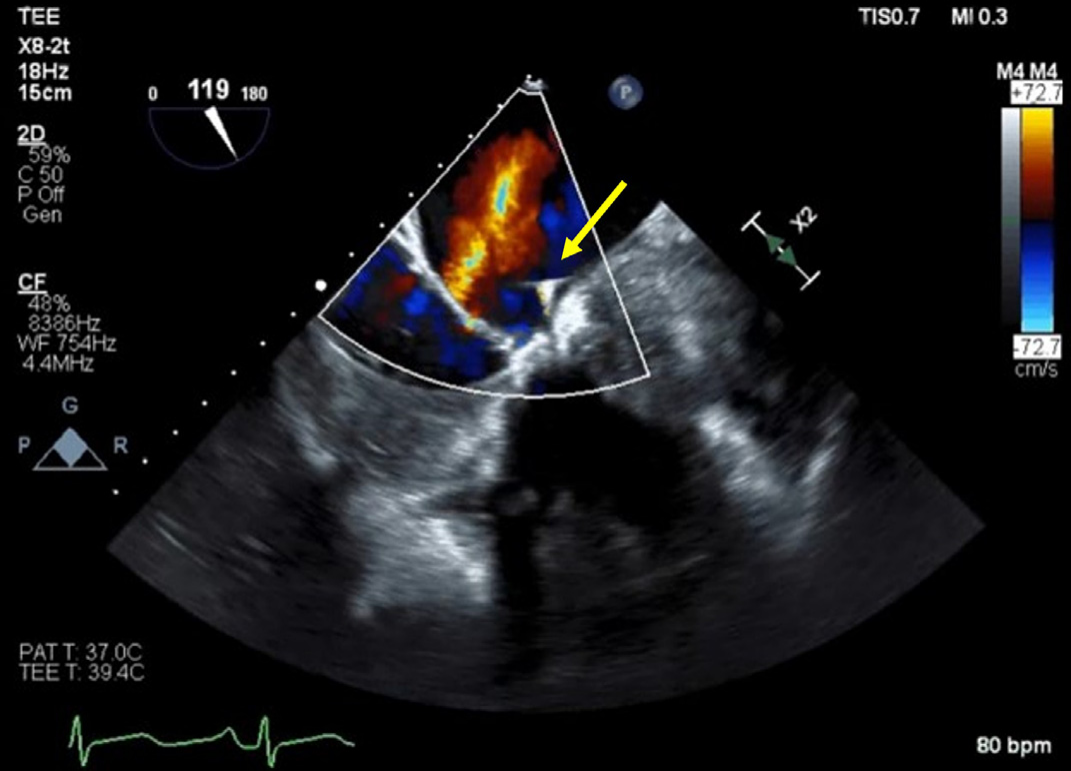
Case 2 Postprocedural transesophageal echocardiography (TEE) showed resolution of mitral regurgitation in the perforated area (yellow arrow, Amplatzer Duct Occluder II plug).
